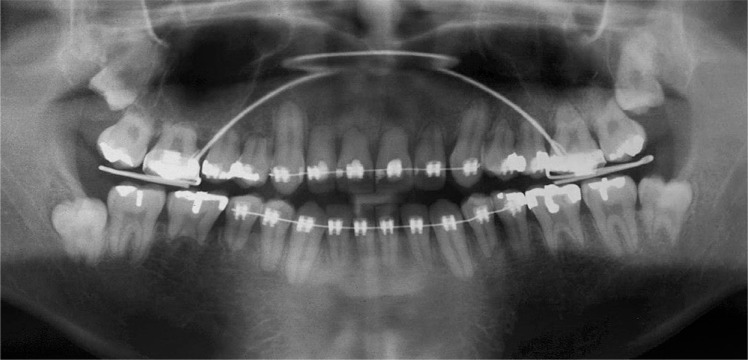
For comparative purposes, note that conventional panoramic radiograph
